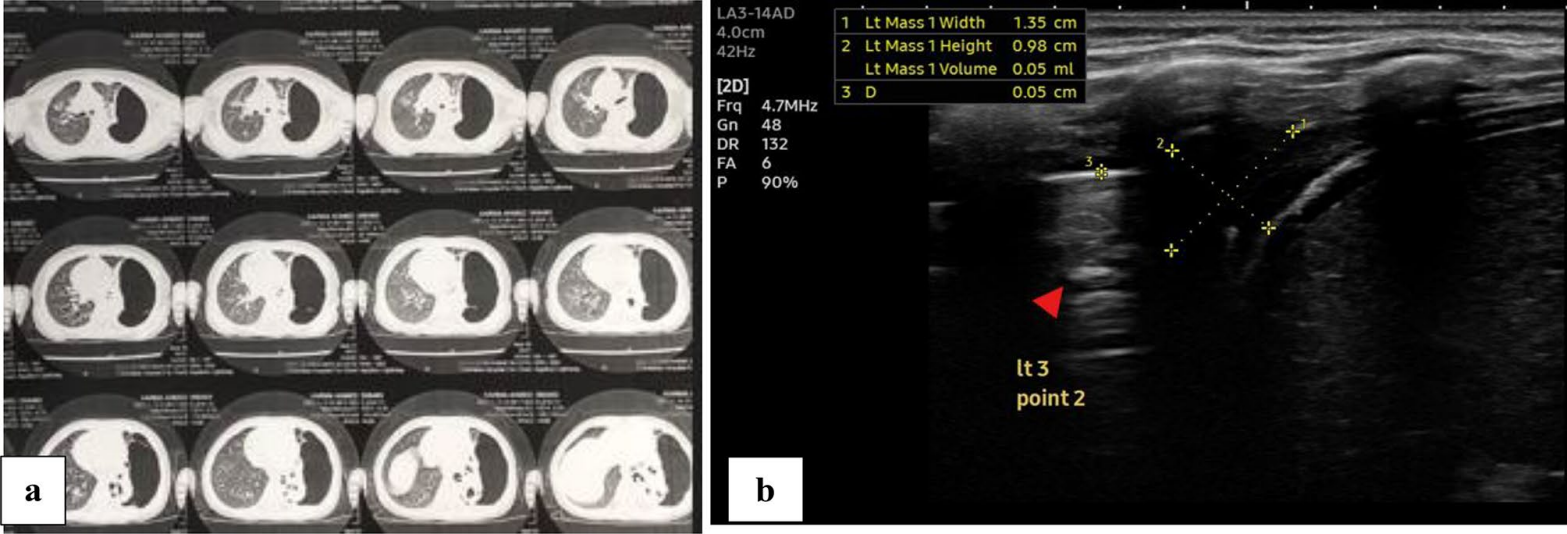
Follow up lung U/S for female patient with complicated community acquired pneumonia. (a) The initial CT done on admission of the patient showing moderate encysted left sided pneumothorax with left lower lobar collapse. (b) The follow up lung U/S done after chest tube insertion showing residual mild right sided pleural effusion with left lower lobar increased echogenicity, loss of the A lines with fluid bronchogram (arrow head)
